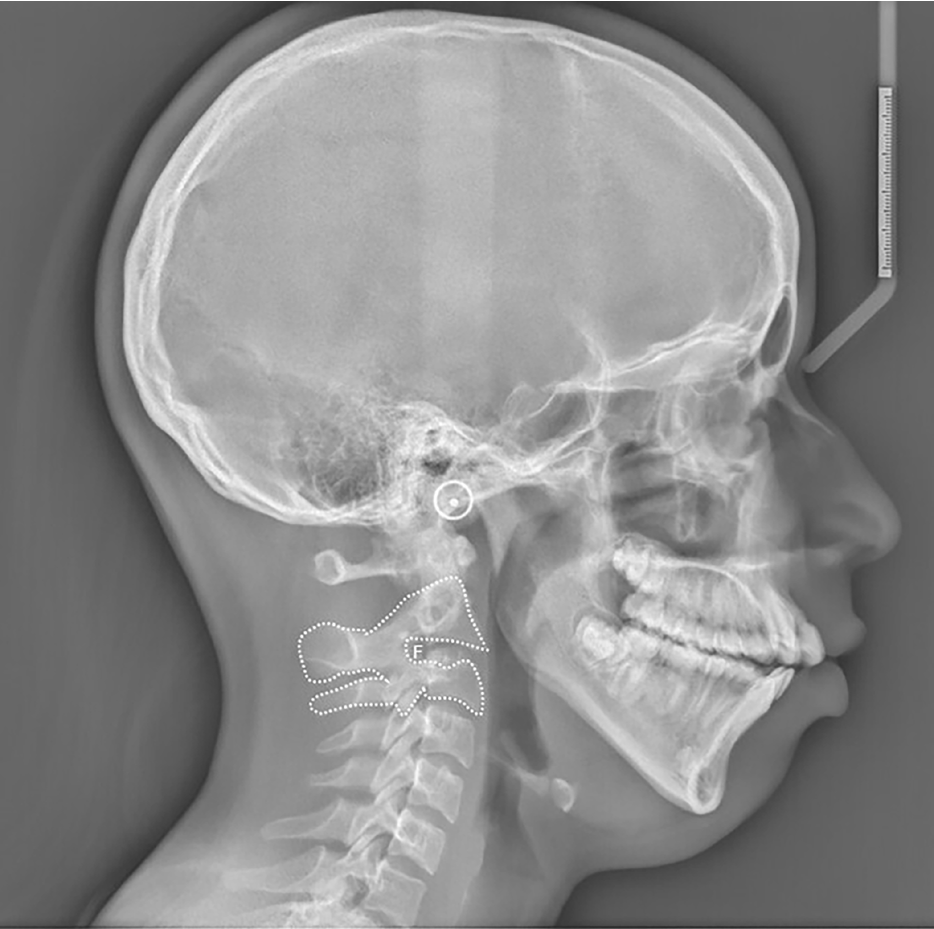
Lateral cephalometric skull radiograph demonstrating the fusion (F) between the C2 and C3 vertebrae.
